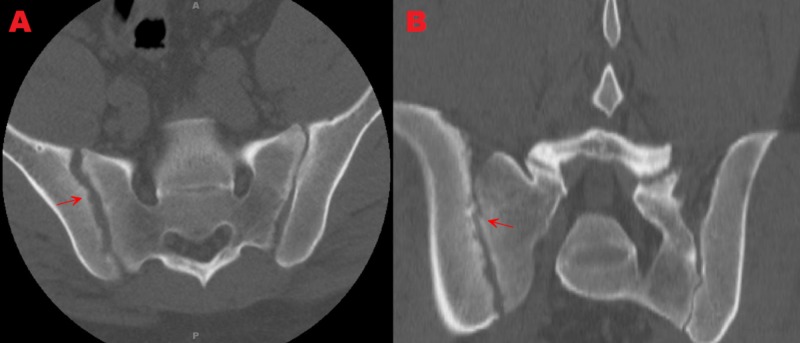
Axial and coronal computed tomography (CT) views showing right sacroiliac joint cortical destruction, erosive changes, and widening. Figure 1a: Axial view showing sacroiliac joint cortical destruction, erosive changes, and widening. Figure 1b: Coronal view showing sacroiliac joint cortical destruction, erosive changes, and widening.
